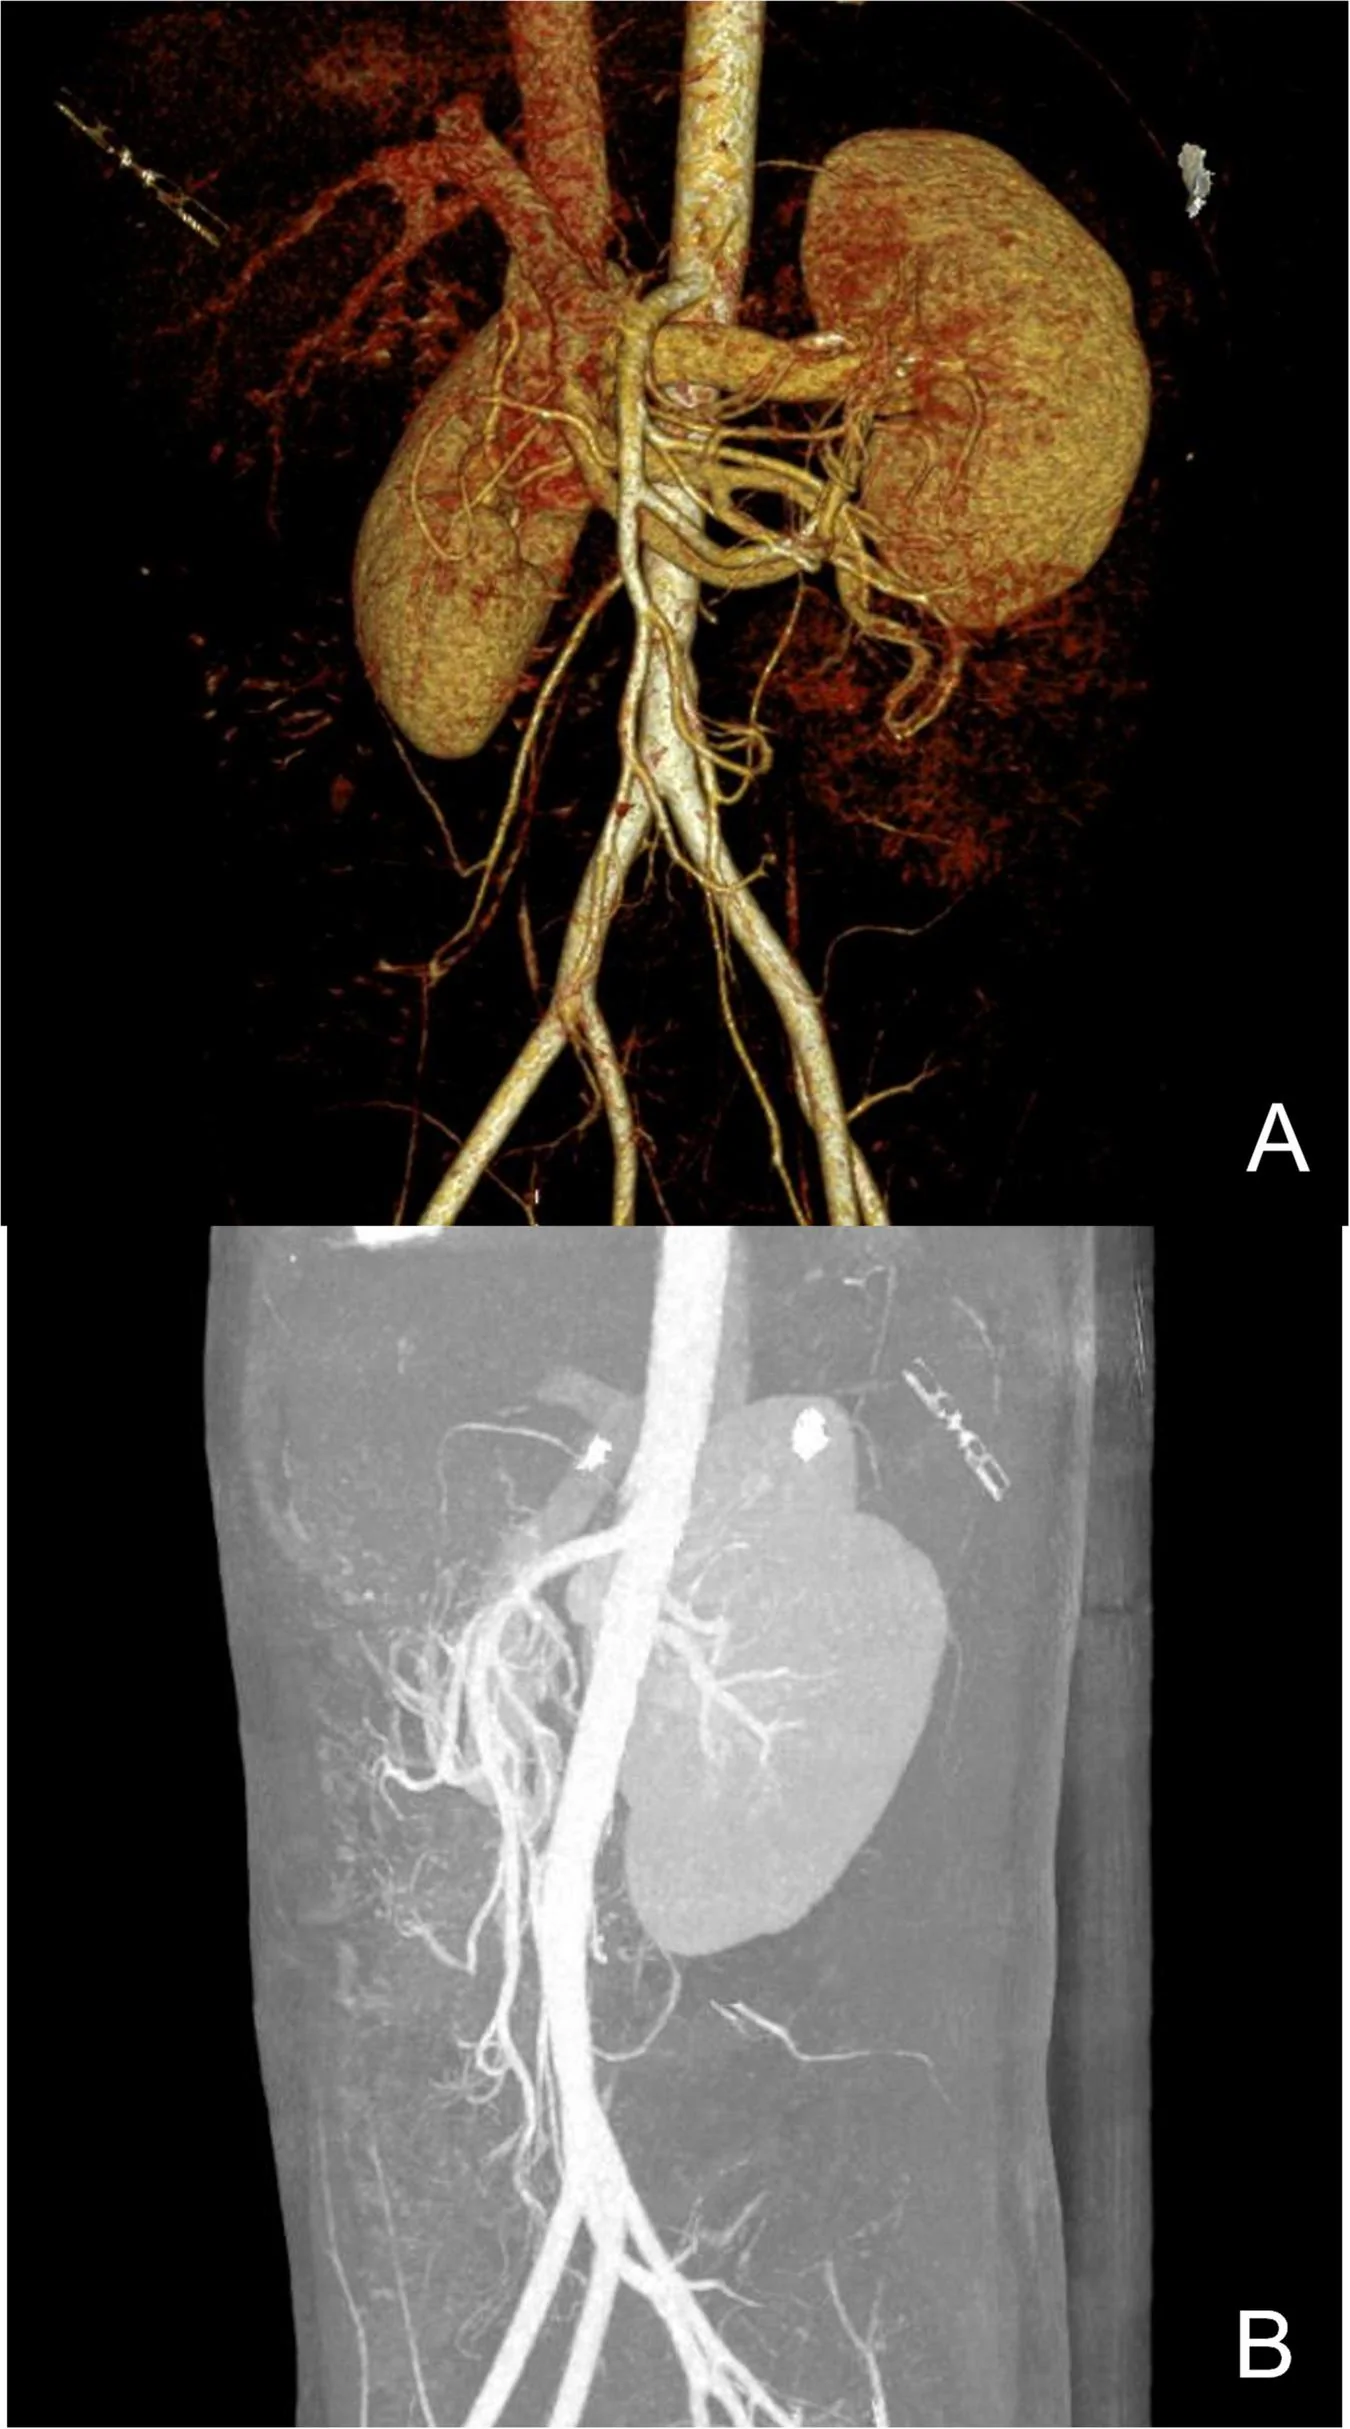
(A, B) Technical artifacts in three-dimensional CTA reconstruction of abdominal vessels. The image is degraded by extensive red flocculent artifacts, resulting in poor delineation of vessel margins and obscuration of key anatomical structures, including the origin of the celiac trunk.
